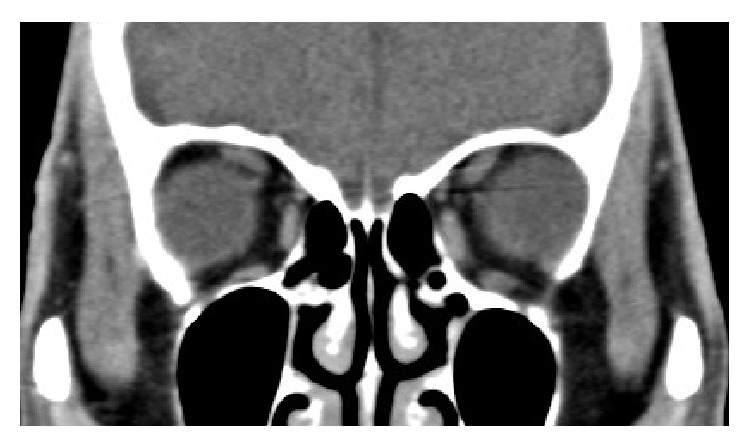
Coronal cut of computed tomography of the orbit of a patient showing superotemporal herniation of the eyeball with inferior displaced LR and nasally displaced SR muscles.
